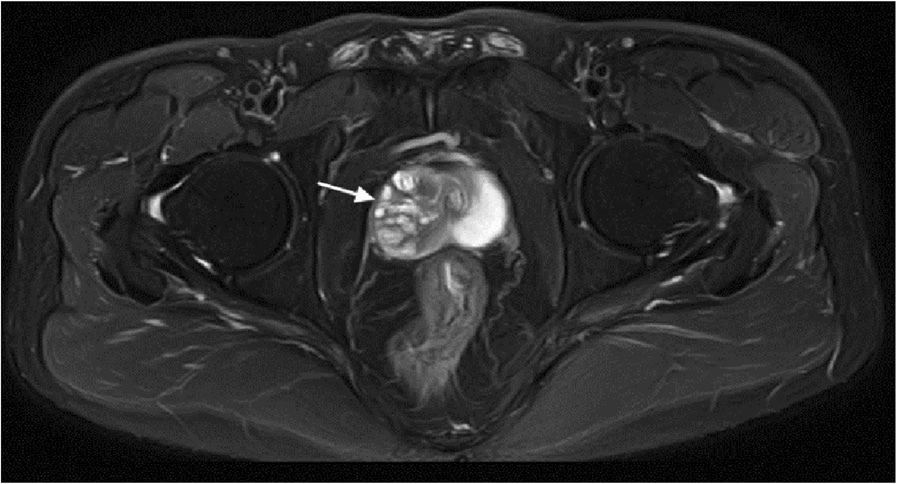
MRI—Transverse section. T2 weighted phase. Multiple nodules of long T2 signal are visible in the right area of the prostate
